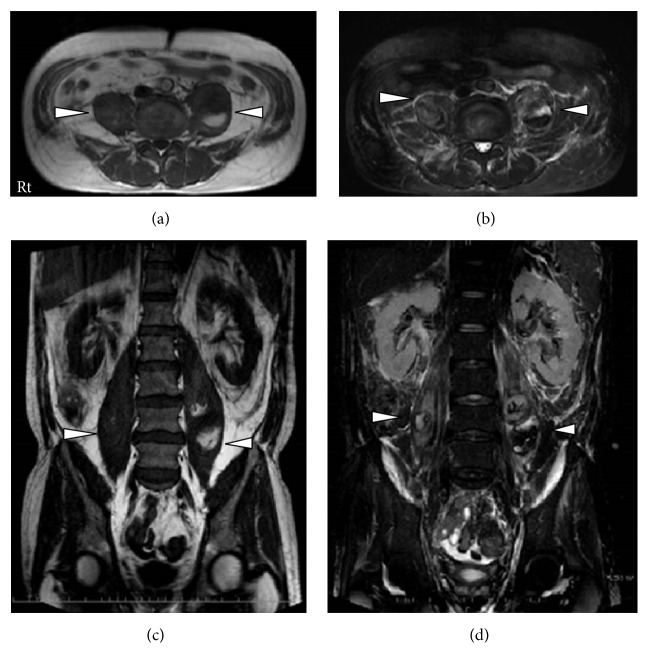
Abdominal magnetic resonance imaging. The bilateral iliopsoas abscesses are indicated by arrow heads. (a) T1-weighted axial image. (b) T2-weighted axial image. (c) T1-weighted coronal image. (d) T2-weighted coronal image.
